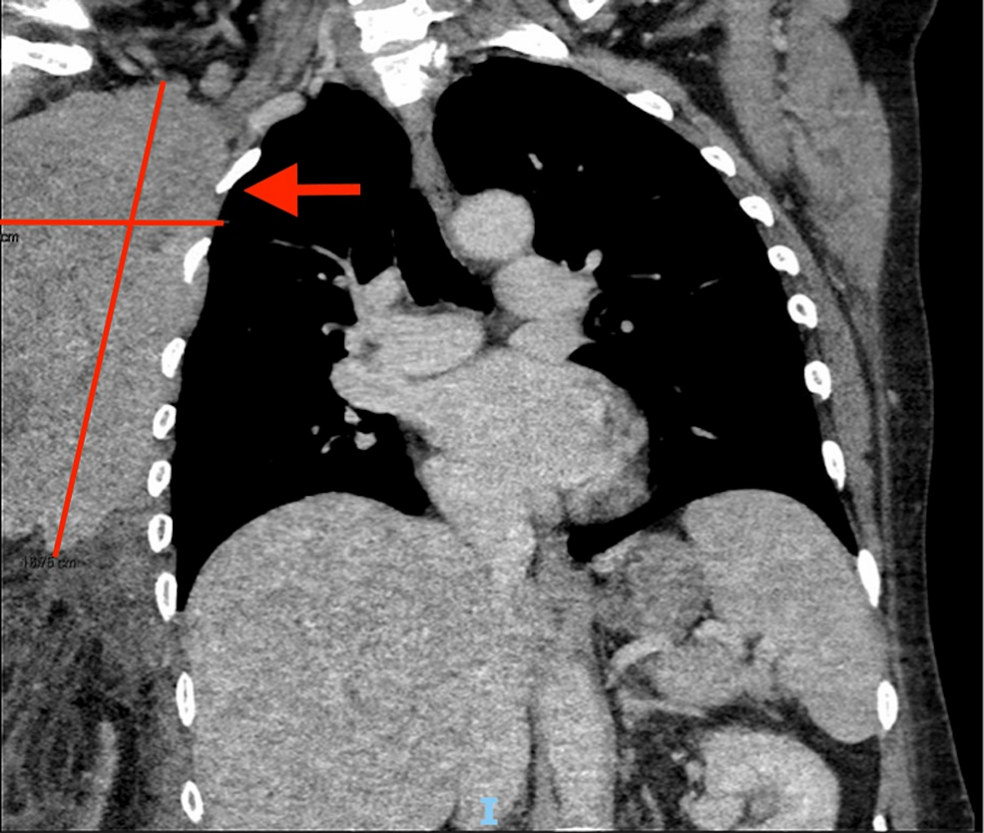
Chest CT scan showed the right breast and an axillary mass of 7x14cm in this plane (arrow)
